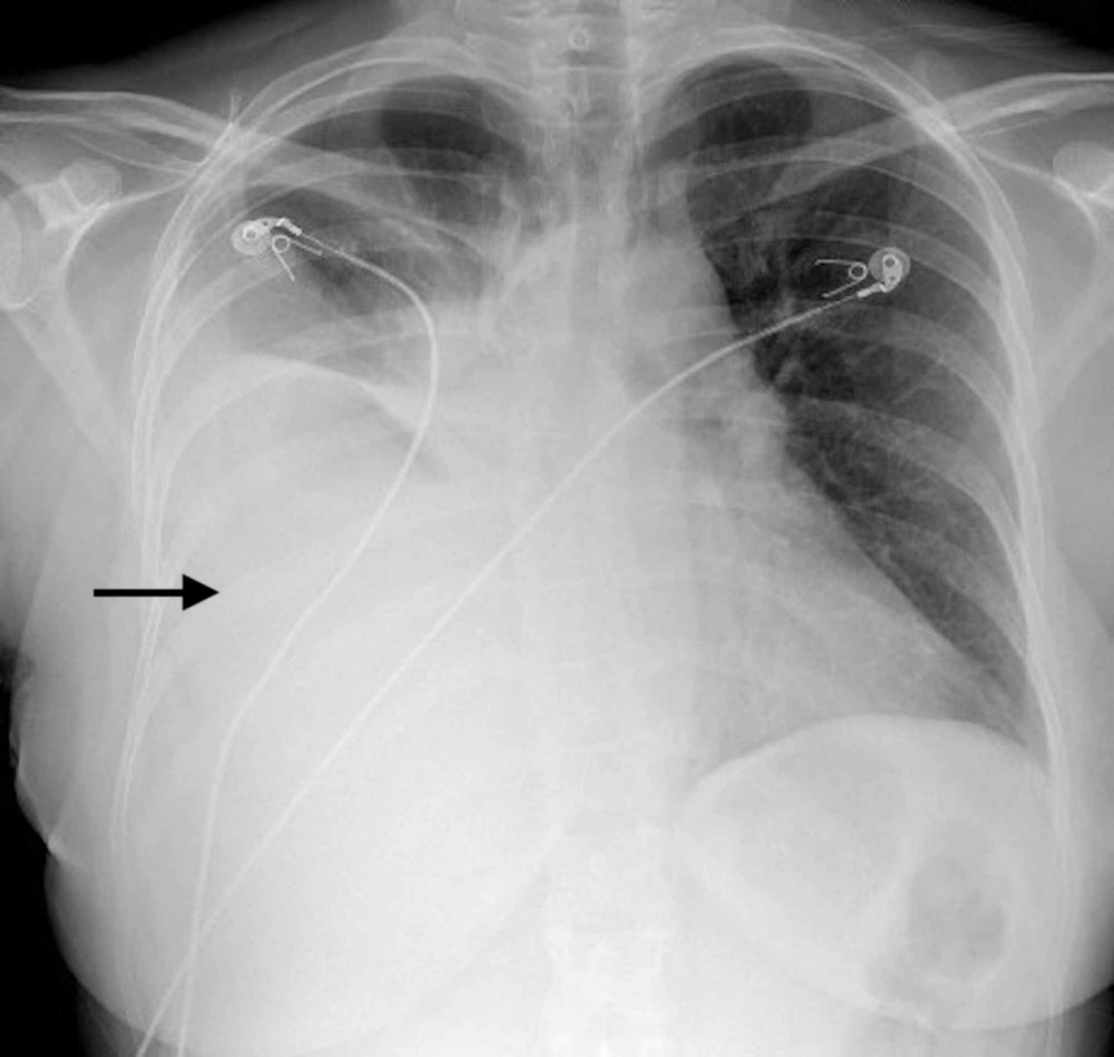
Chest X-ray with arrow pointing to a large right-sided pleural effusion
